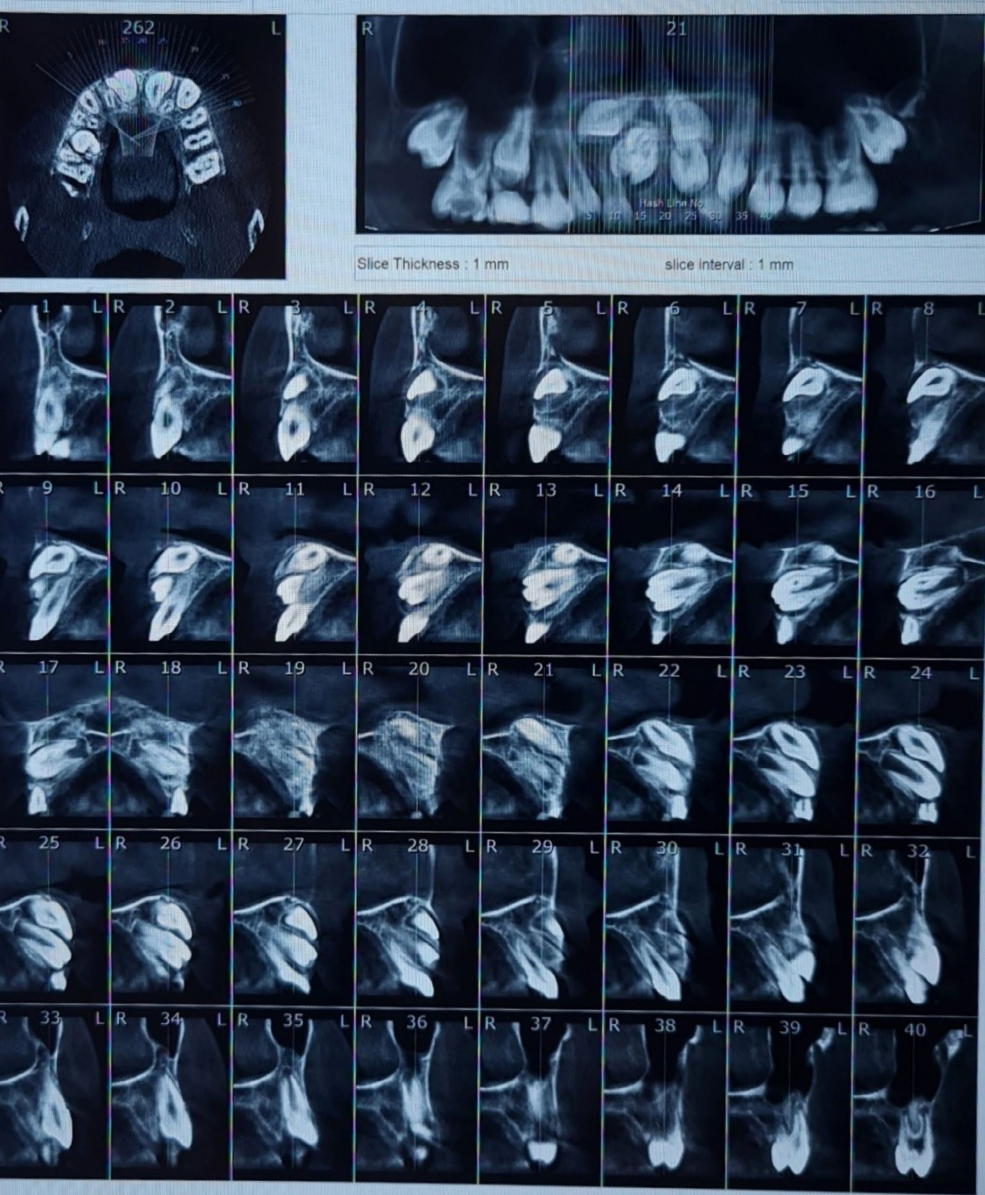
CBCT (axial, coronal, sagittal) demonstrates enamel‐lined invagination within each supernumerary tooth (mesiodens), consistent with DI, and their 3D relationship to the unerupted incisors. These findings—indistinct on panoramic imaging—identify the precise obstruction pathway and invagination depth (Oehlers's classification), directly informing surgical timing and approach.
